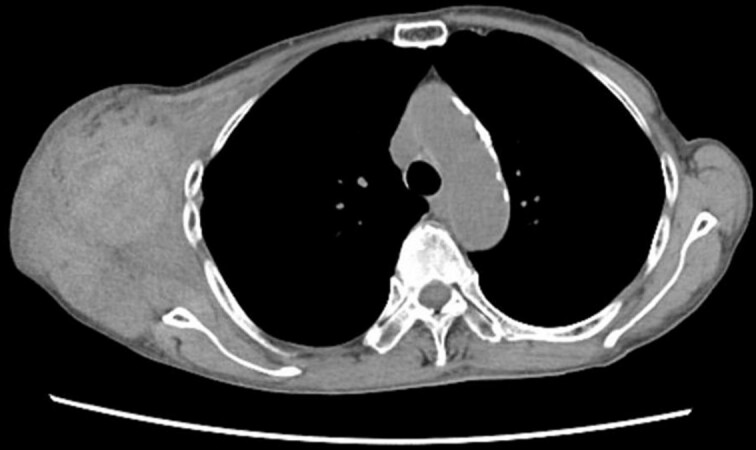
Chest CT at the time of the first bleeding showed formation of a hematoma on the right chest and front of the scapula.
